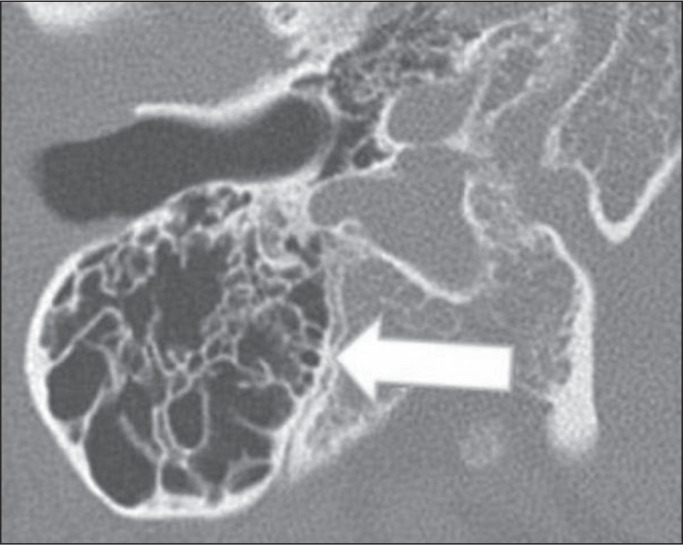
Occipitomastoid suture in a 10-year-old boy. Unenhanced axial CT
